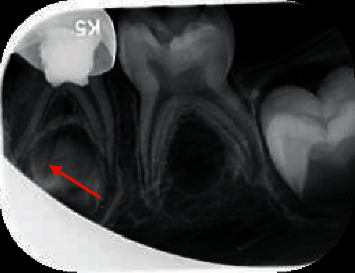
Periapical radiograph showing third root in left mandibular first primary molar.
